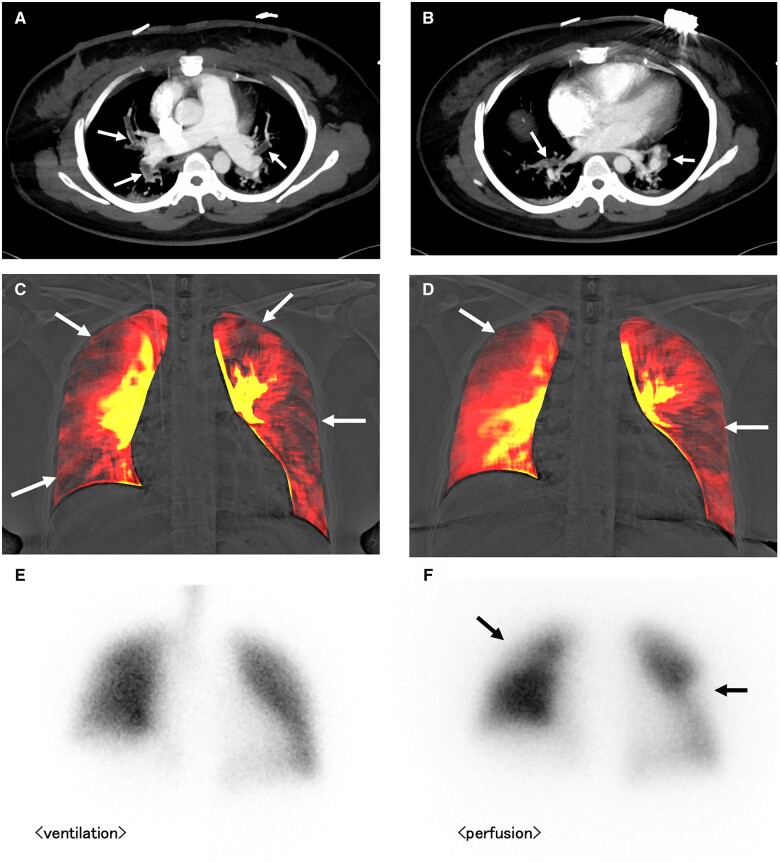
CTEPH is a relatively rare but serious complication after acute PTE. Although V/Q scintigraphy is recommended to detect residual perfusion abnormalities, it is not frequently used. DCR is a novel cineradiography technique using a flat-panel detector and a pulsed X-ray generator that shows pulmonary perfusion from temporal change in X-ray attenuation without any contrast media or radionuclide. The radiation exposure is much lower than that for V/Q and computed tomography scans. Moreover, it can be performed efficiently in outpatient settings. Conversely, the patient’s inability to keep still or to hold his or her breath for 7–10 s could lower the accuracy of the study. This is the first report that shows DCR-based detection of progression of acute PTE to CTEPH.
